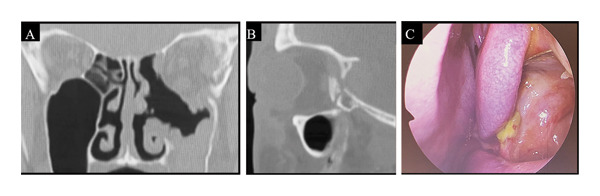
Postoperative CT and endoscopy image. (A, B) CT images taken 1 week postoperatively. All bone fragments had been removed, and decompression of the orbit had been achieved. (C) Left nasal 0‐degree endoscopy image taken 1 month after surgery. Epithelialization of the maxillary sinus was good. CT: computed tomography, ILPPL: inferolateral periorbital periosteal line.
